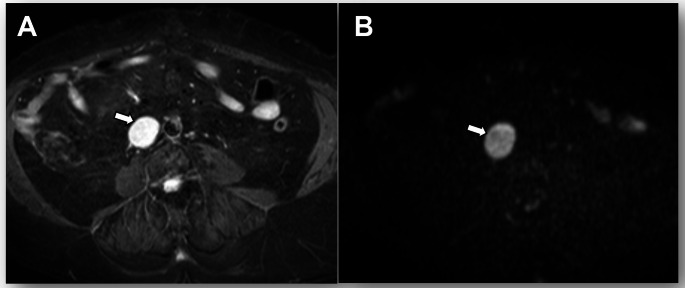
Stage IIIC2 grade 2 endometrioid carcinoma in a 63-year-old woman. A Axial fat-suppressed T2-weighted fast spin-echo (FSE) image (TR/TE: 4500/100 ms; slice thickness: 3 mm) shows an enlarged para-aortic lymph node (arrow). B Axial diffusion-weighted image (DWI) with b = 800 s/mm^2^ shows a hyperintense lymph node (arrow). ADC value in the node is 0.94 × 10^−3^ mm^2^/s. Pathological analysis confirmed lymph node metastases
